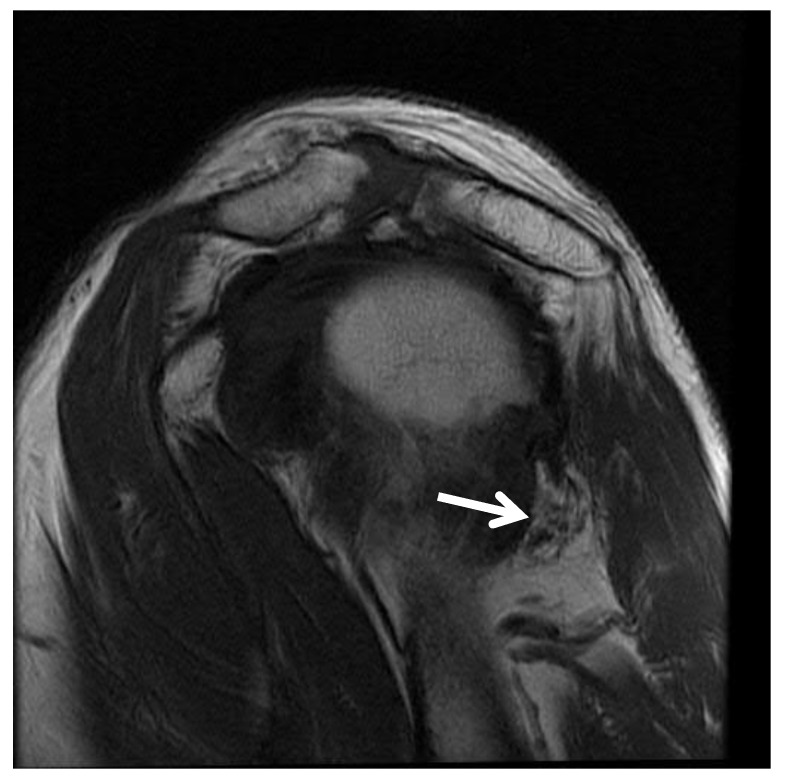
Atrophic left teres minor on magnetic resonance imaging (MRI) of shoulder in patient with quadrilateral space syndrome.
